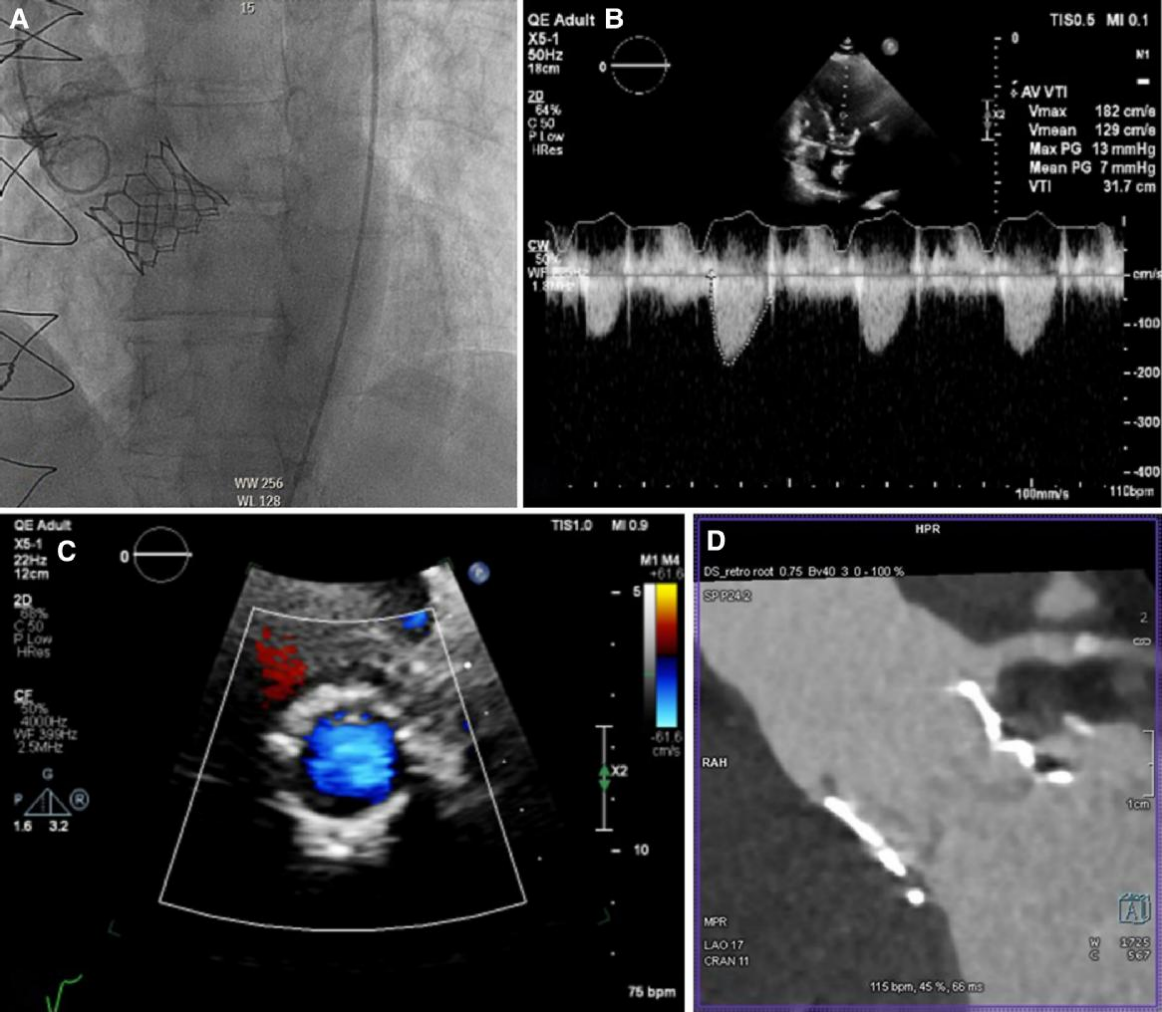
(*A*) Post-transcatheter aortic valve replacement aortography showed no aortic regurgitation: (*B*) continuous wave Doppler through the aortic valve; (*C*) colour Doppler in the short axis view; (*D*) computed tomography at follow-up showed preservation of left main coronary access.
